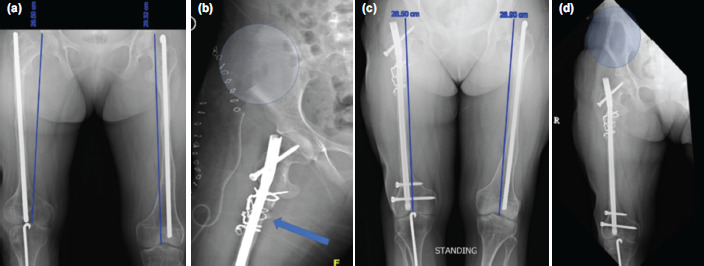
(a) Pre-operative radiograph with estimated 3cm shortening and malrotation evidence by obliquely overlapped distal femoral condyle, patella facing laterally, and the fibula lies behind the tibia. (b) Post-operative radiograph showing bone graft at the subtrochanteric region stabilised with cerclage wire (arrow) with large area of tricortical graft defect (circle). (c) Post-operative radiograph with well incorporation of the bone graft with acceptable length. (d) Healing of the iliac donor site (circle) and well incorporated femur graft.
